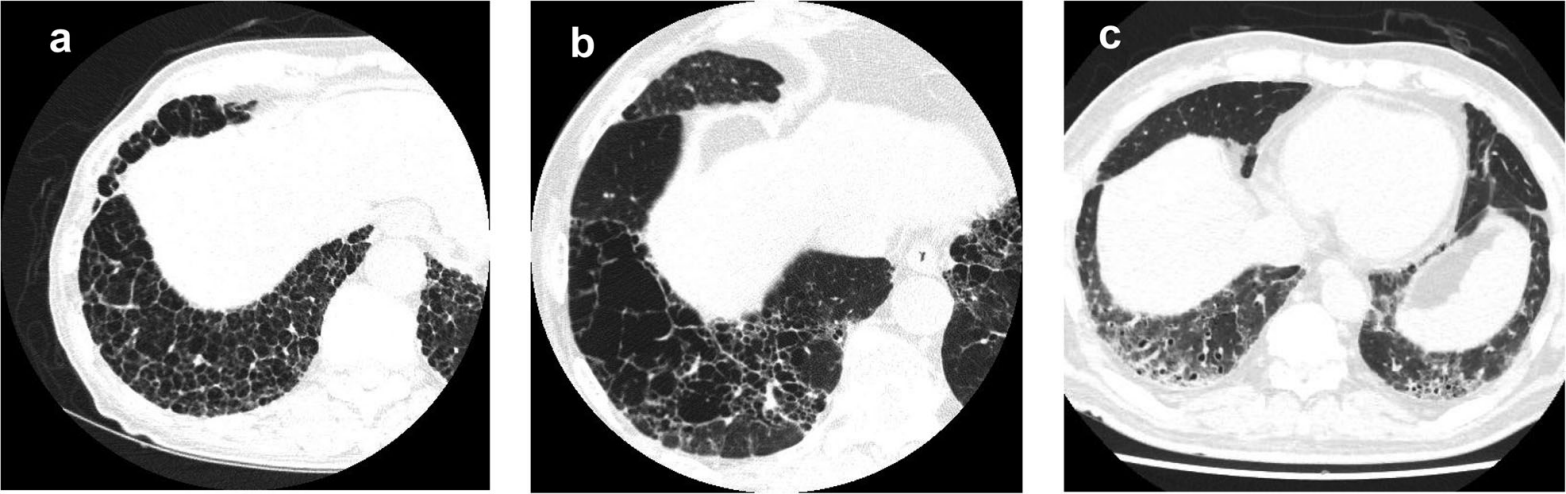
Background lung findings before treatment with pembrolizumab. (a) Computed tomography (CT) of the lung field in Case 1. Honeycomb changes were observed immediately below the pleura, and the patient was considered to have usual interstitial pneumonia (UIP). (b) CT image of Case 3. Reticular shadows and emphysema were observed mainly in the lower lobe. The honeycomb structure is not clear. This was determined to be possible UIP pattern. (c) CT image of Case 8. Reticulated shadow and extensive ground‐glass opacities (GGOs) were observed mainly in the dorsal lower lobe of both lungs. This was determined to be inconsistent with UIP pattern.
